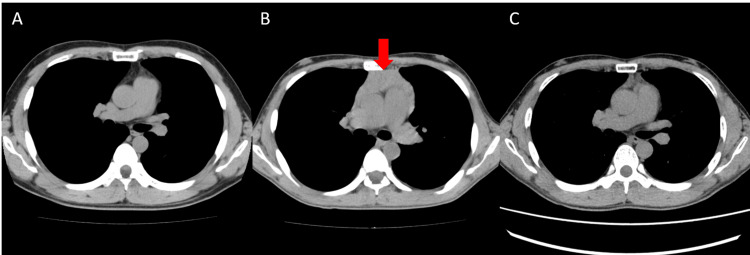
Changes over time in the thymic hyperplasia on chest CT scans A: Two months before the onset of thyrotoxicosis symptoms. B: Three months after the onset of thyrotoxicosis symptoms, the thymic was enlarged. C: Seven months after surgery, the thymic hyperplasia had decreased on chest CT. CT: computed tomography
